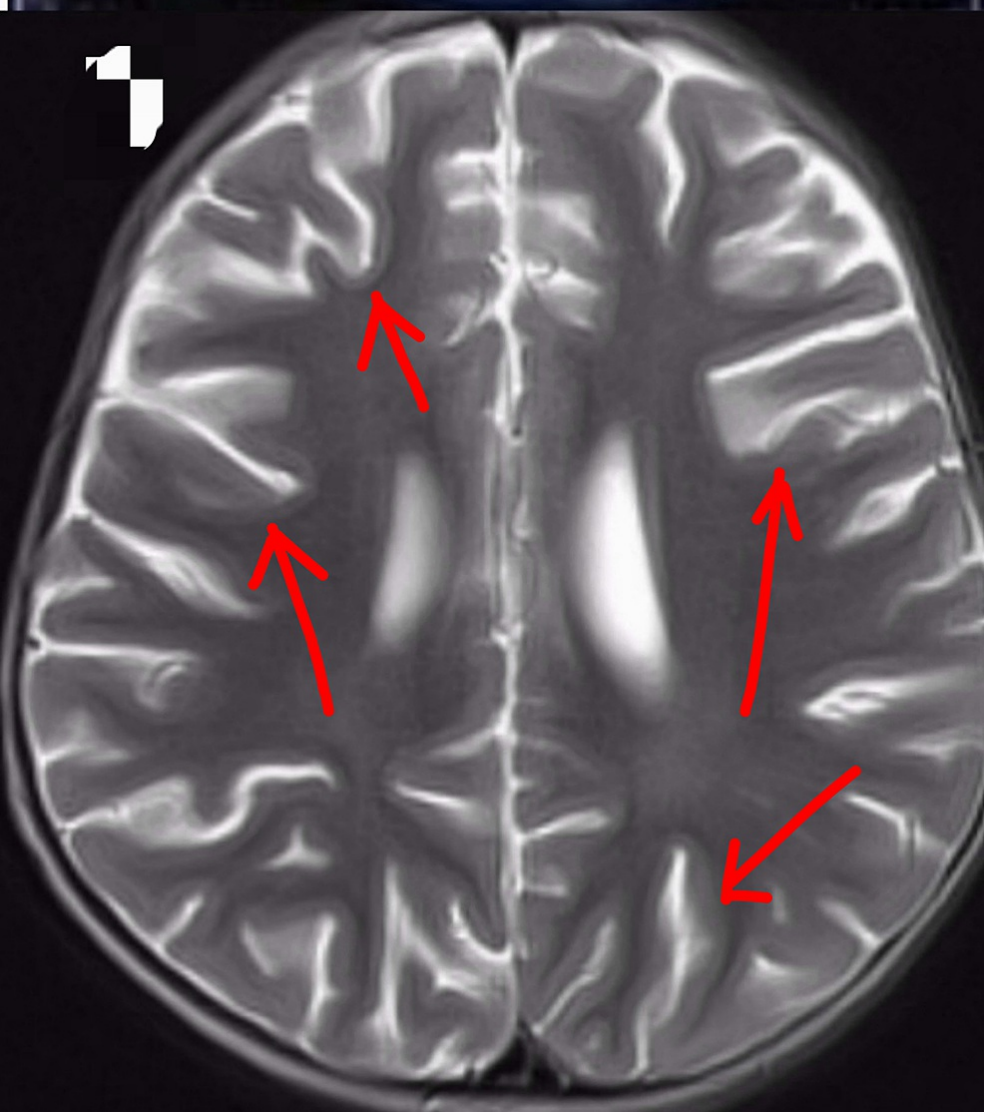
Magnetic resonance image of the brain T2 axial sequence showing features of cerebral atrophy.
